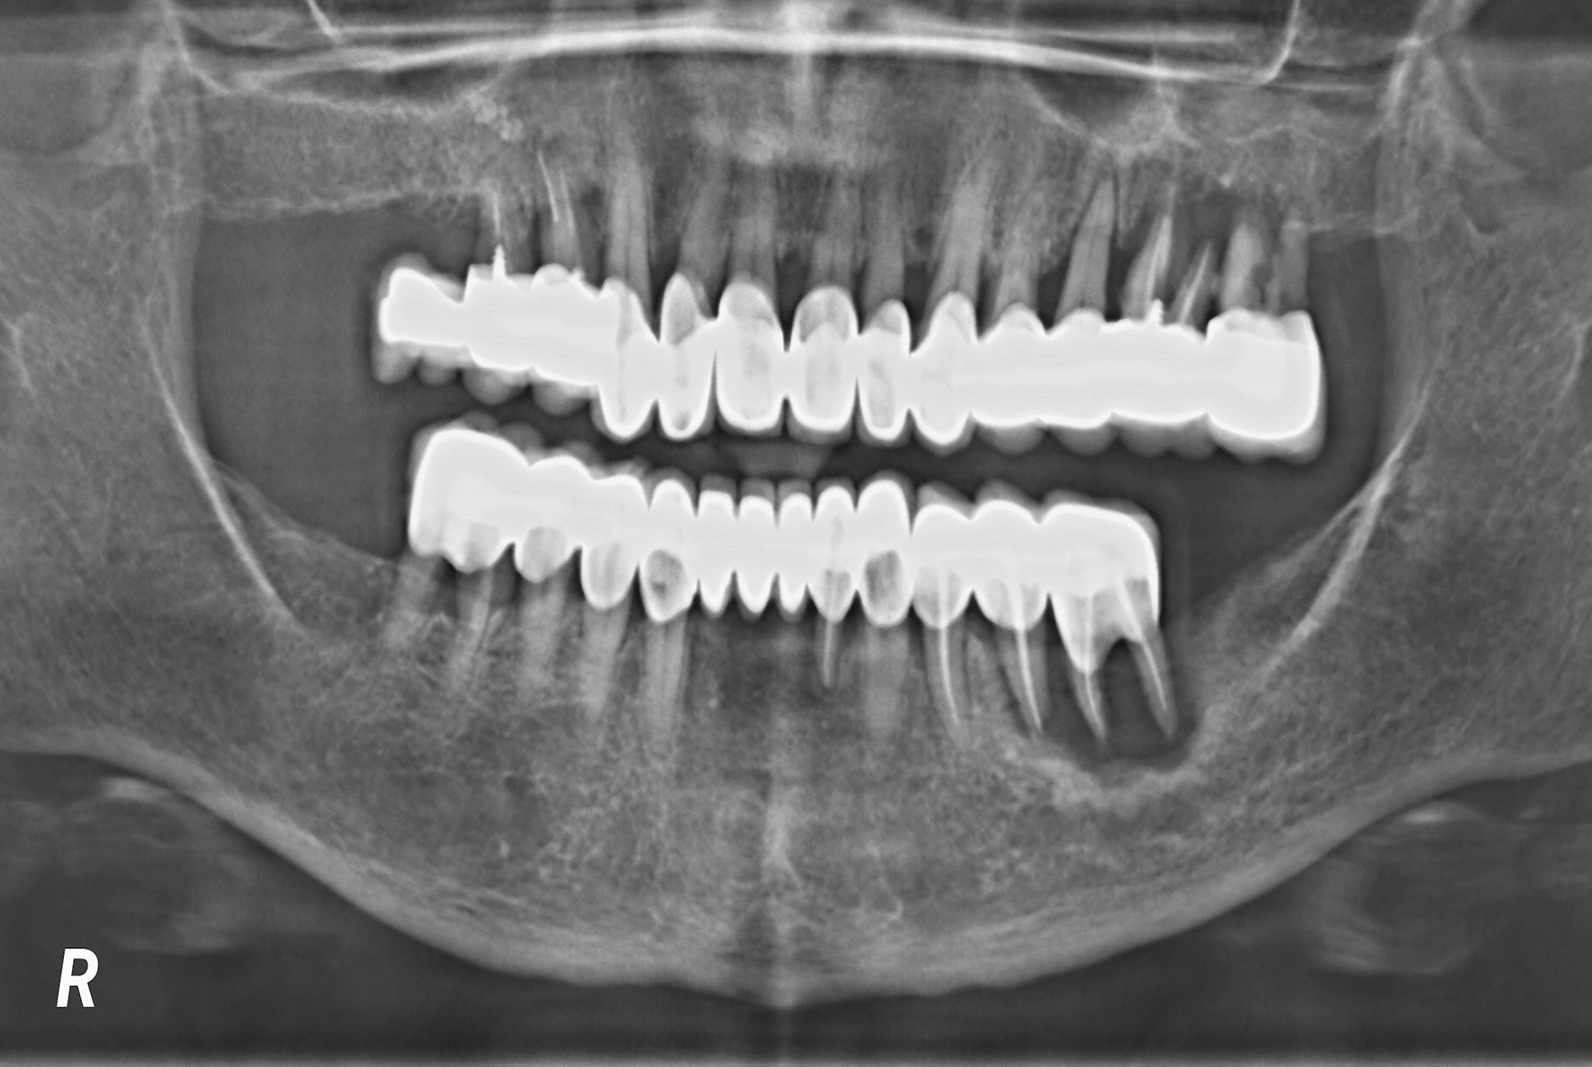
Panoramic X-ray showing bone loss around the posterior teeth for a patient having long-span fixed prosthesis
